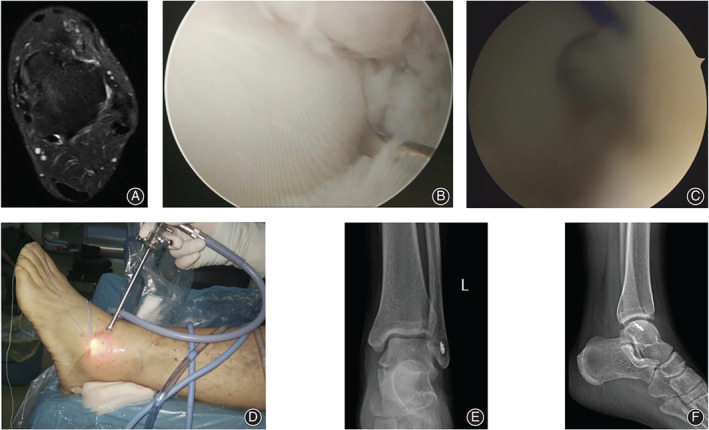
A 28‐year‐old male patient with chronic lateral ankle instability for 15 months after failure of 8 months of conservative treatment. (A) The preoperative MRI showed the integrity of the ATFL was interrupted. (B) The intra‐operative view under the arthroscope showed the ATFL detachment at the fibular side, and the laxity of the ATFL was recorded after probe palpation. (C) A suture passer was used to pass the anchor arms through the ATFL and inferior extensor retinaculum. (D) The anchor arms were sutured by horizontal mattress suture fashion. (E) The postoperative anterior–posterior X‐ray film of the operated ankle. (F) The lateral X‐ray film of the left ankle after the surgery.
